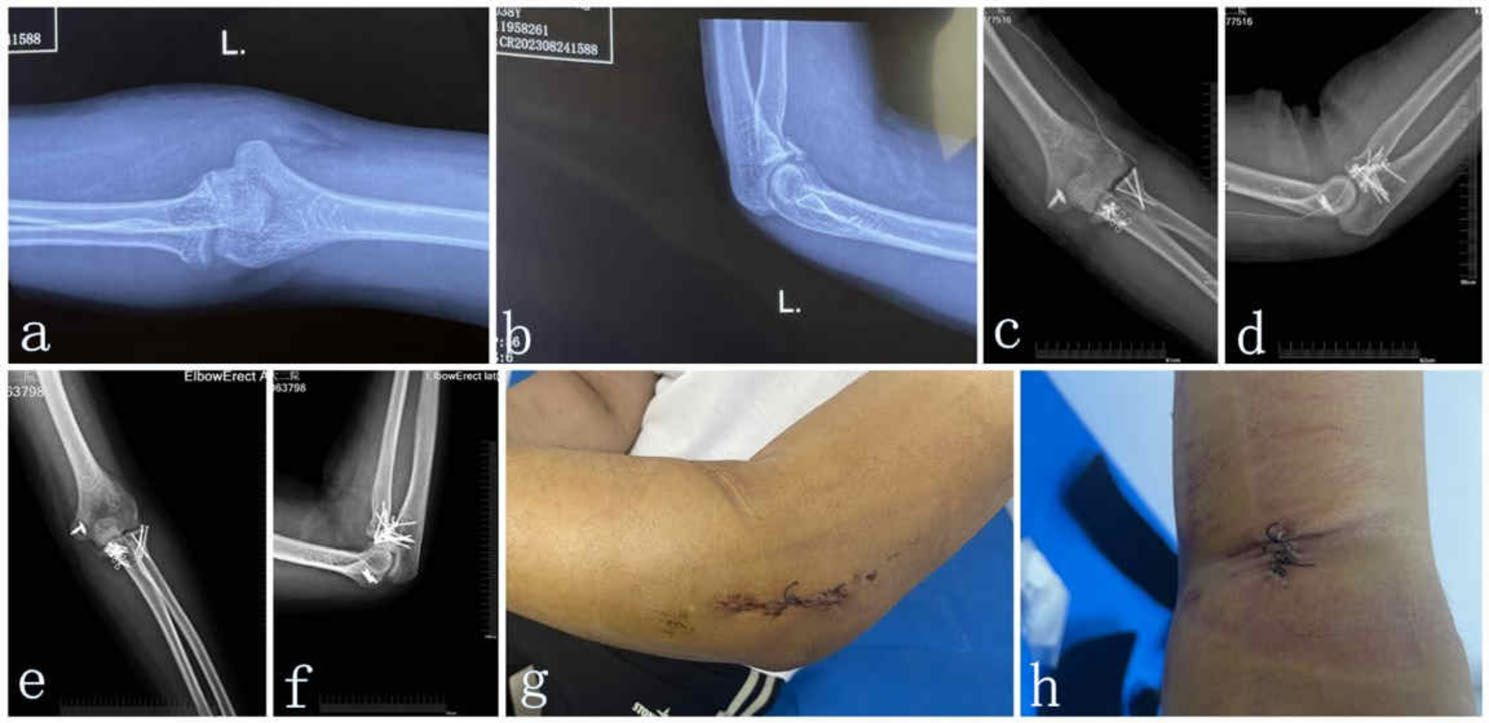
Representative “terrible triad injury of elbow”. (a, b) Preoperative radiographs. (c, d) Immediately postoperative radiographs. (e, f) After three months the operation. (g, h) The incision of the operation
